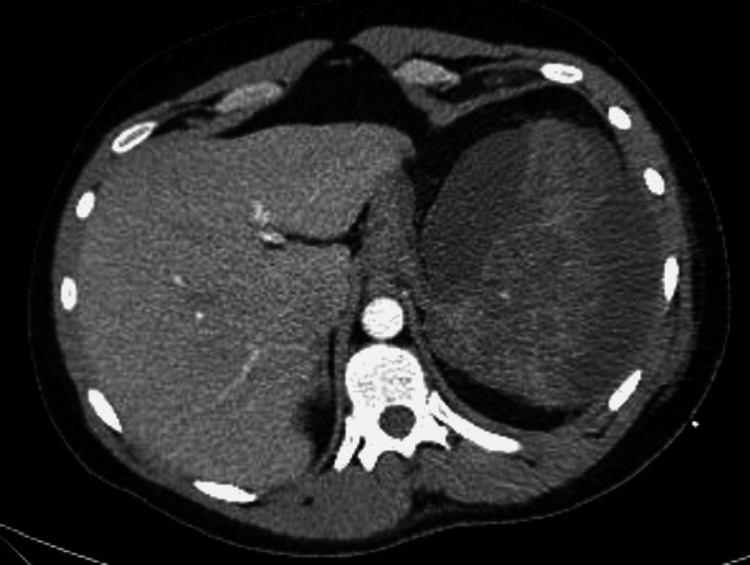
Subsequent computed tomography of the abdomen and pelvis showing the progression of splenic infarction with significant perisplenic fluid on day nine.
